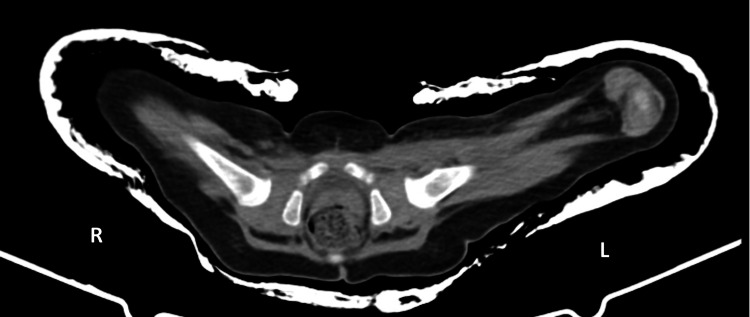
Postreduction axial CT scan confirming concentric reduction of the femoral head within the acetabulum. R: right. L: left
